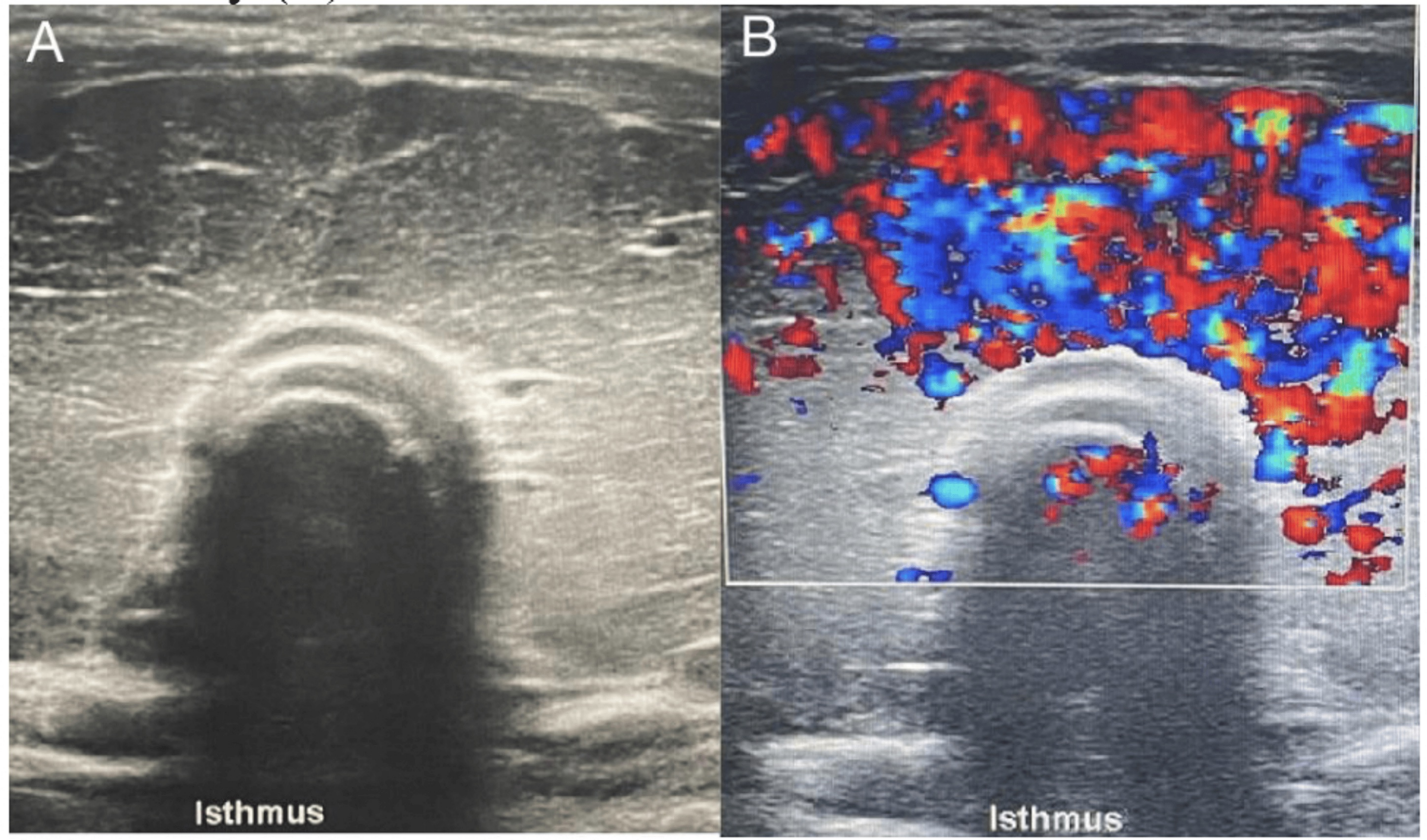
Thyroid ultrasound showing diffuse enlargement of both lobes of the thyroid gland with coarse, heterogeneous parenchymal echotexture (A). Color Doppler shows enhanced vascularity (B). N/A
